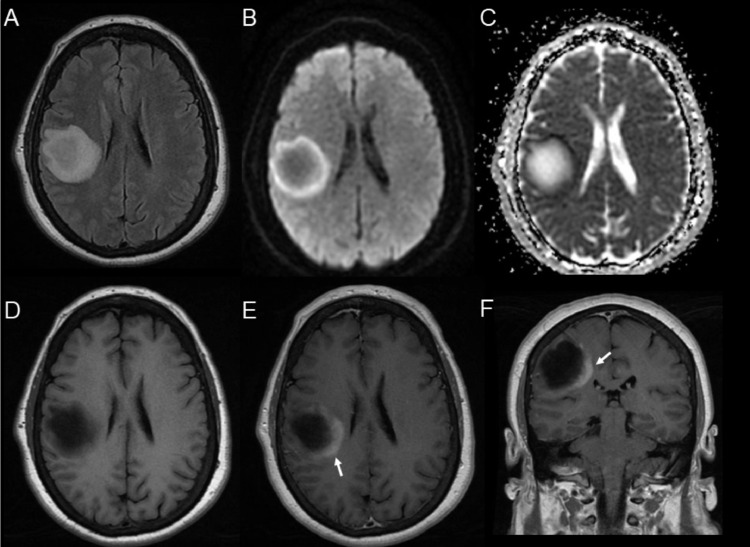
Initial MRI demonstrating a right frontoparietal mass with partial ring-enhancement and mild local mass effect. Axial brain MRI fluid inversion recovery imaging (A), diffusion weighted imaging (B) with apparent diffusion coefficient (C), T1-weighted (D), and gadolinium-enhanced axial (E) and coronal (F) images show a right frontoparietal mass, which measures approximately 4.7 cm in maximum diameter. A mild degree of edema and minimal mass effect are present. A ring of restricted diffusion (B, C) and incomplete ring-enhancement can be seen (E, F, arrows).
